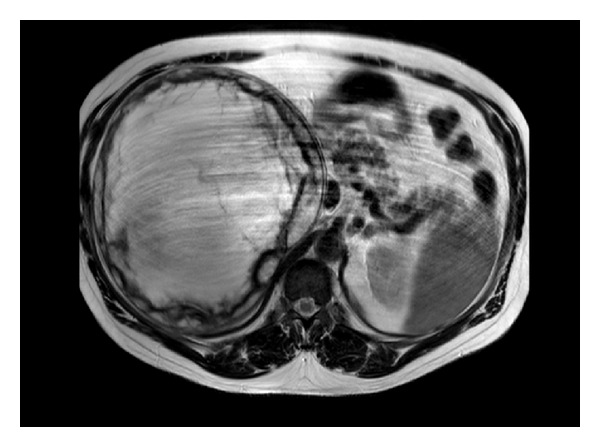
Patient's MRI T2-weighted image with heterogeneously low signals.
